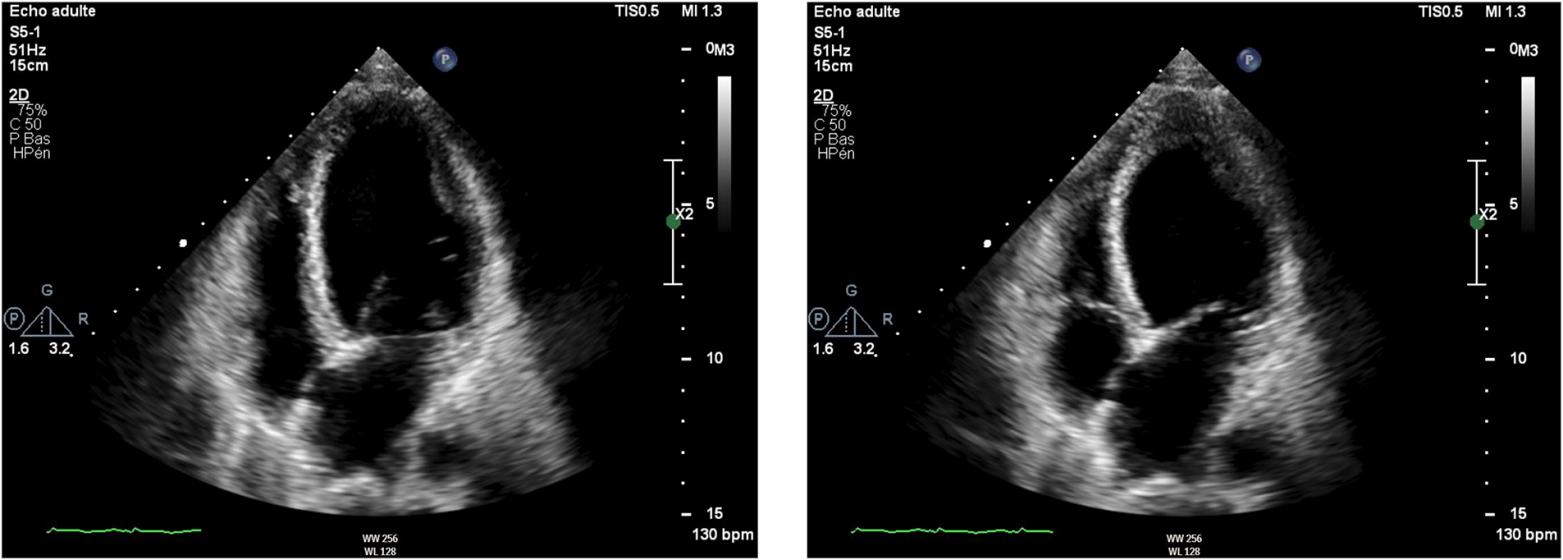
Transthoracic echocardiography performed at day one, showing a severely reduced left ventricular ejection fraction (24%) with basal and midventricular segments akinesia, and preserved apical contraction. There was no significant valvulopathy and right ventricular function was normal. A: Diastole, B: Systole.
